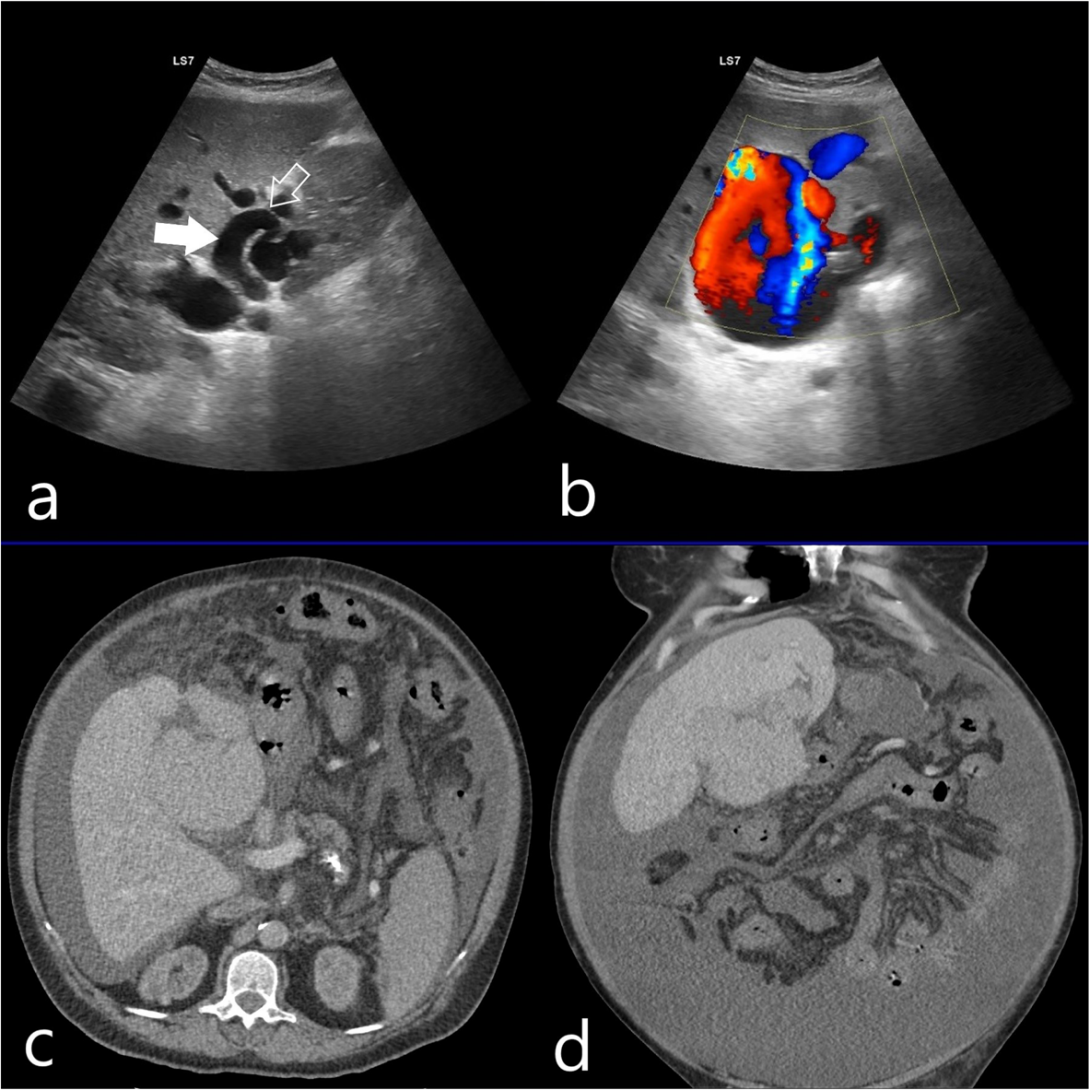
RDUS view (a and b) showing the direct connection (hollow white arrow) between dilated hepatic artery (solid white arrow) and portal vein (a); Large saccular aneurysm formation between left hepatic artery and portal vein (b). Portal phase CT (c,d) confirms US findings and demonstrates massive ascites
